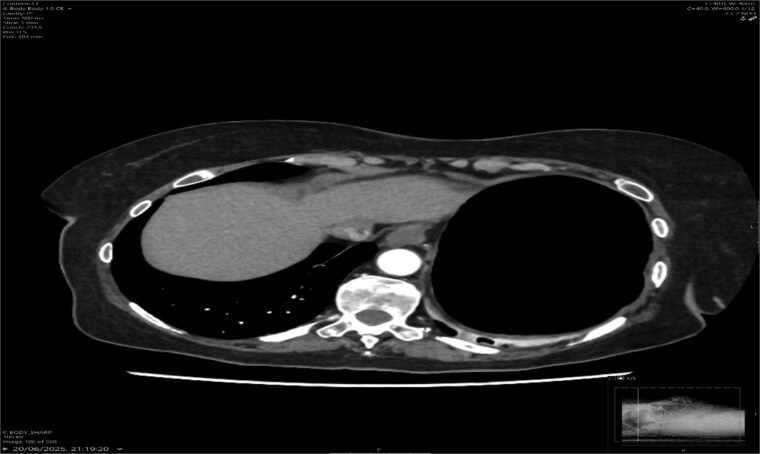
Axial CT showing dilated transverse colon at the splenic flexure.
